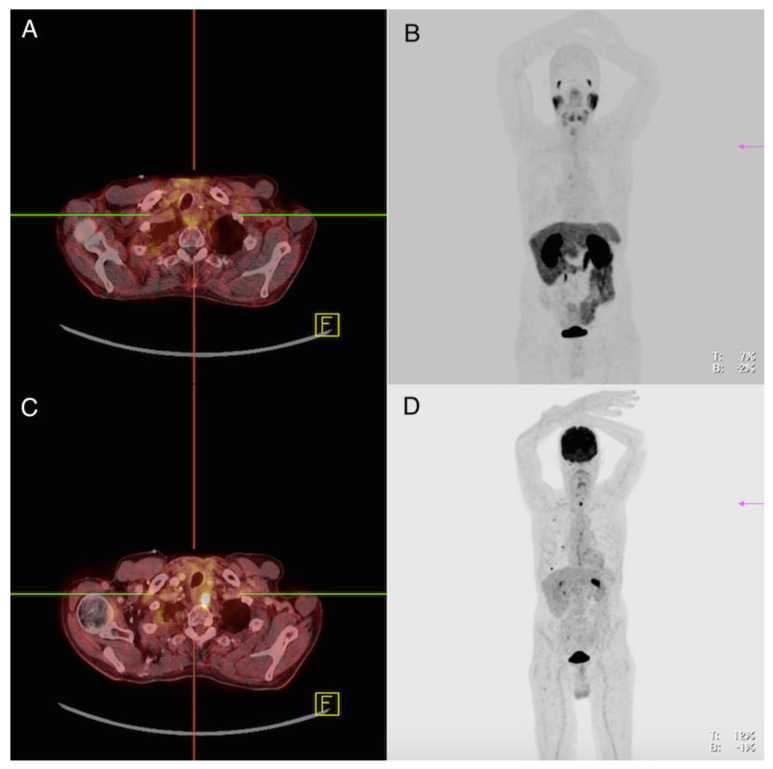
Patient 1. ^68^Ga-PSMA-PET/CT (A,B), ^18^FDG-PET/CT (C,D). The finding was compatible with a local recurrence in the left thyroid bed. The FDG-positive lesion showed no PSMA expression.
